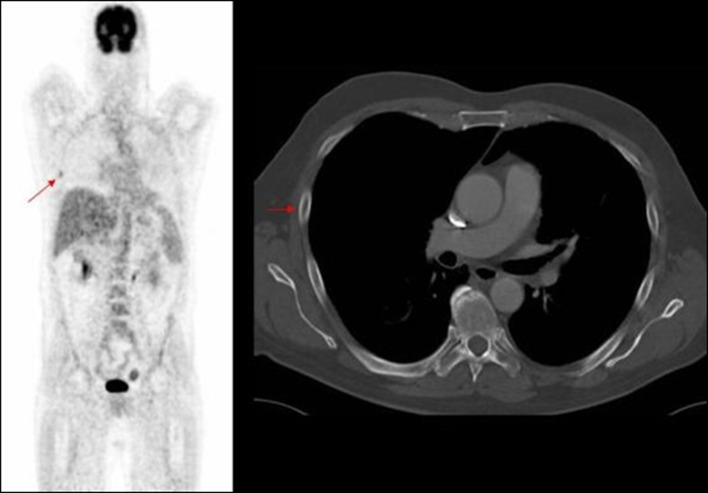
PET image of rib deposit initially noted in PET, not identified on corresponding axial CT image.
